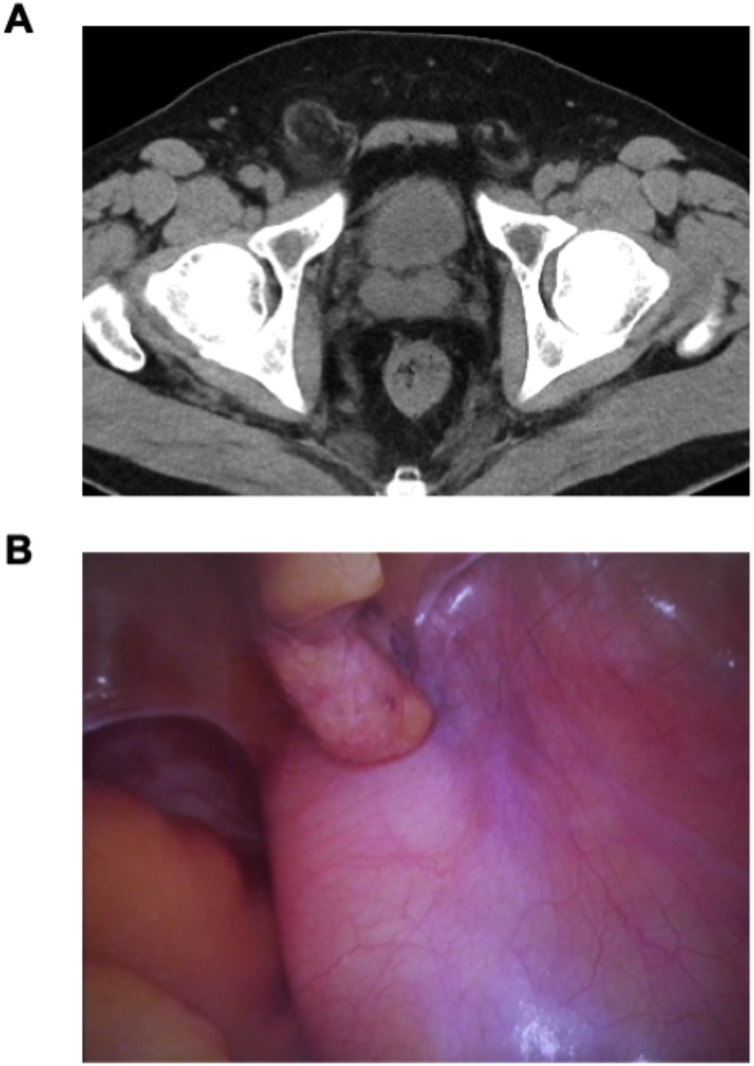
(A) Non-contrast computed tomography suggested an inguinal herniation. (B) Laparoscopic image of swollen retroperitoneal soft tissues.
